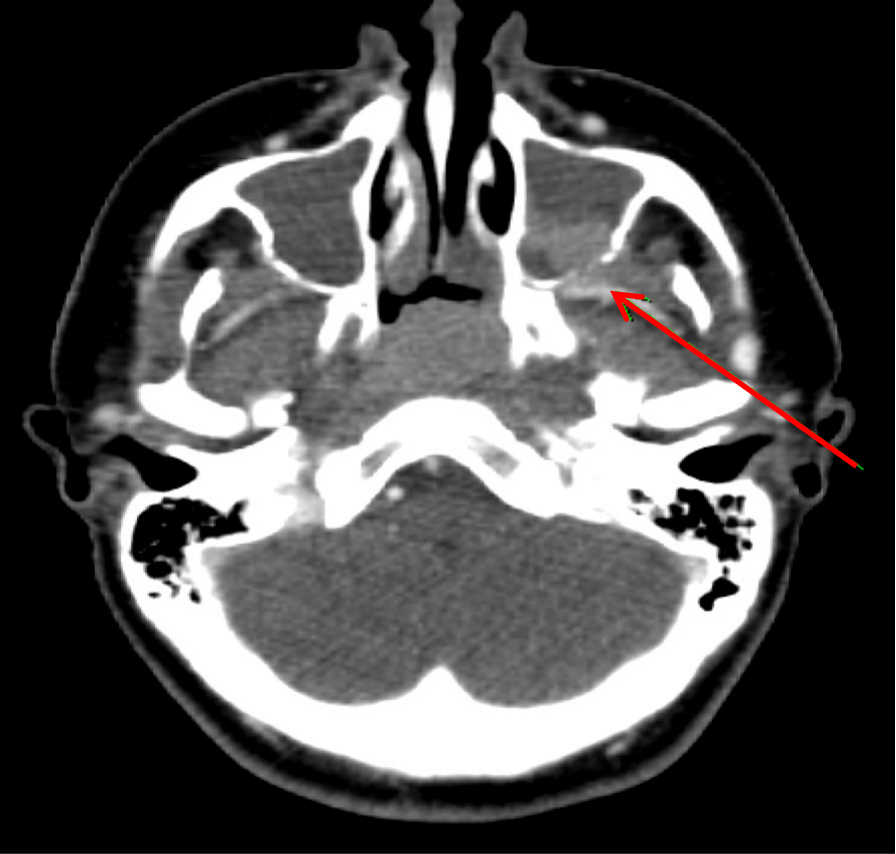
Head CT with contrast of a 16-year-old male who developed a nasopharyngeal carcinoma 3.3 years after starting infliximab infusions
